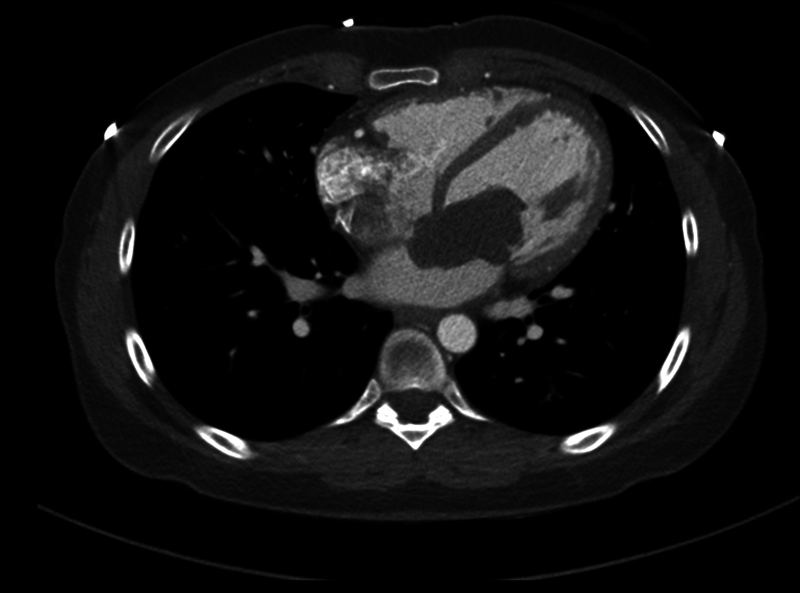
A computed tomography axial view demonstrating the huge left atrial mass protruding to the left ventricle through the mitral valve.
